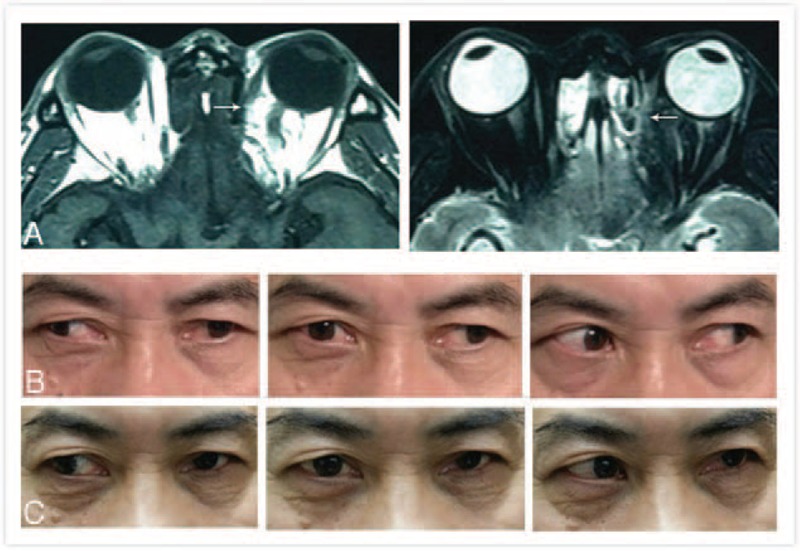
A–C: Preoperative and postoperative alignment and magnetic resonance imaging (MRI) of case 36 with a total rupture of the left medial rectus muscle after sinus surgery. A: MRI in both T1WI and T2WI demonstrates the total rupture present in the left medial rectus muscle. B: Before surgery, the patient had a 50 PD left exotropia and a scale of −4 motility defect of left medial rectus muscle. C: 6 months after strabismus surgery (9 mm resection of the ruptured medial rectus muscle and 12 mm recession of the antagonist lateral rectus muscle), orthotropia in primary position and a scale of −3 and −2 motility defect of both the left medial rectus and lateral rectus muscles, respectively. MRI = magnetic resonance imaging, PD = prism diopter. .
